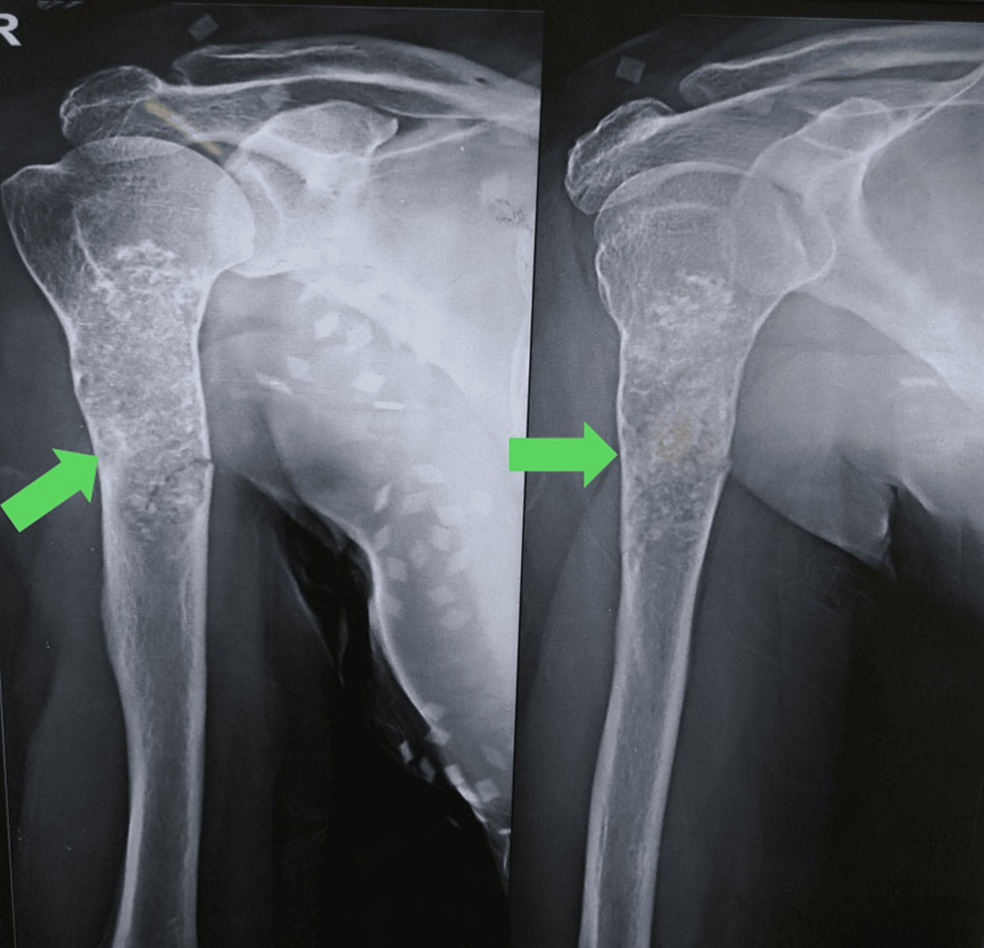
Pre-operative radiographs showing chondrosarcoma involving part of the humerus
